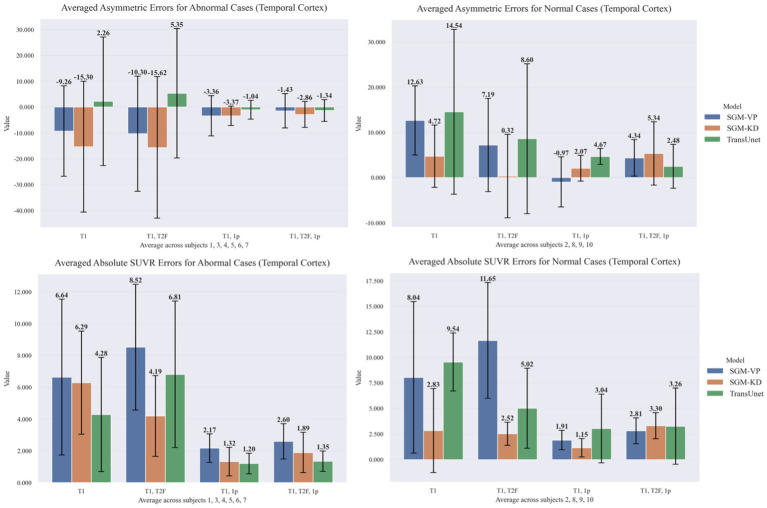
Visualizations of temporal lobe cortex specific asymmetric errors and SUVR errors for the normal cases and abnormal cases.
